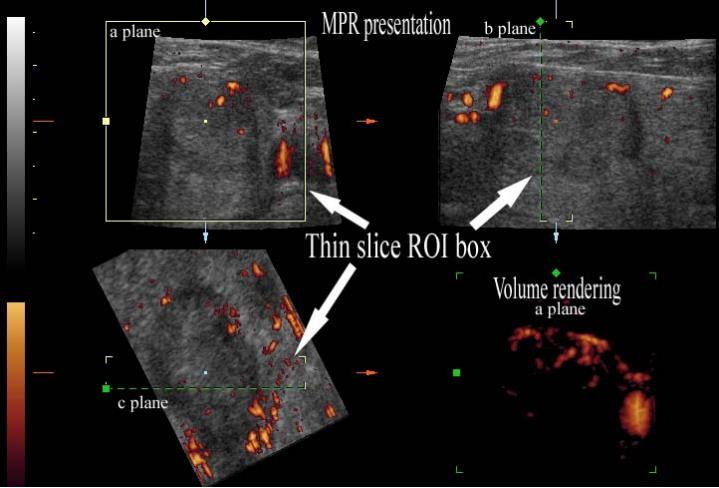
Three-dimensional power Doppler ultrasound of papillary cancer with low density of vessels. Multiplanar reformation (MPR) mode presentation and thin-slice volume rendering (lower right corner). The maximal density of the vessels was evaluated on the volume-rendered image (in the a-plane). The borders of the cancer on the a-plane image in MPR were correlated with the distribution of vessels on the volume-rendered image. The density of the vessels is in the lowest range: 1 = (1-25%) of area of the nodule; ROI - region of interest.
